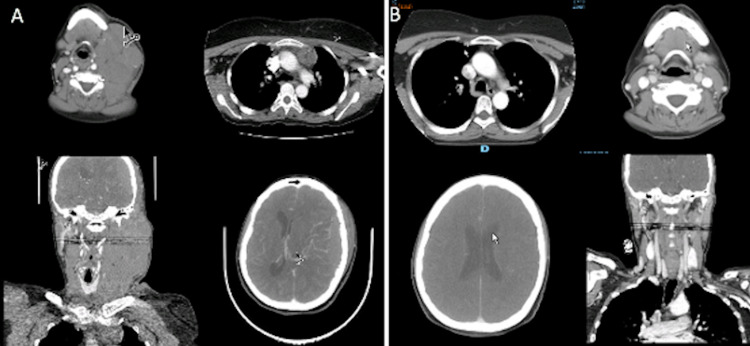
Comparative CT head and neck and brain MRI (A) CT of the neck and chest (October 2017): a mass with a neoplastic appearance occupying the left hemicneck, extending towards the cranial vault, and compromising the epidural space, with a mass effect on the brain parenchyma and subfalcine herniation. Prevascular and bilateral axillary lymph node involvement. Multiple adenomegalies in the right hemicneck. (B) Contrast brain MRI (September 2018): little residual-like dural enhancement in the left parietal location with no evidence of lesions suggesting residue or tumor recurrence. When compared to the initial study on October 31, 2017, the patient is considered to have a complete response.
